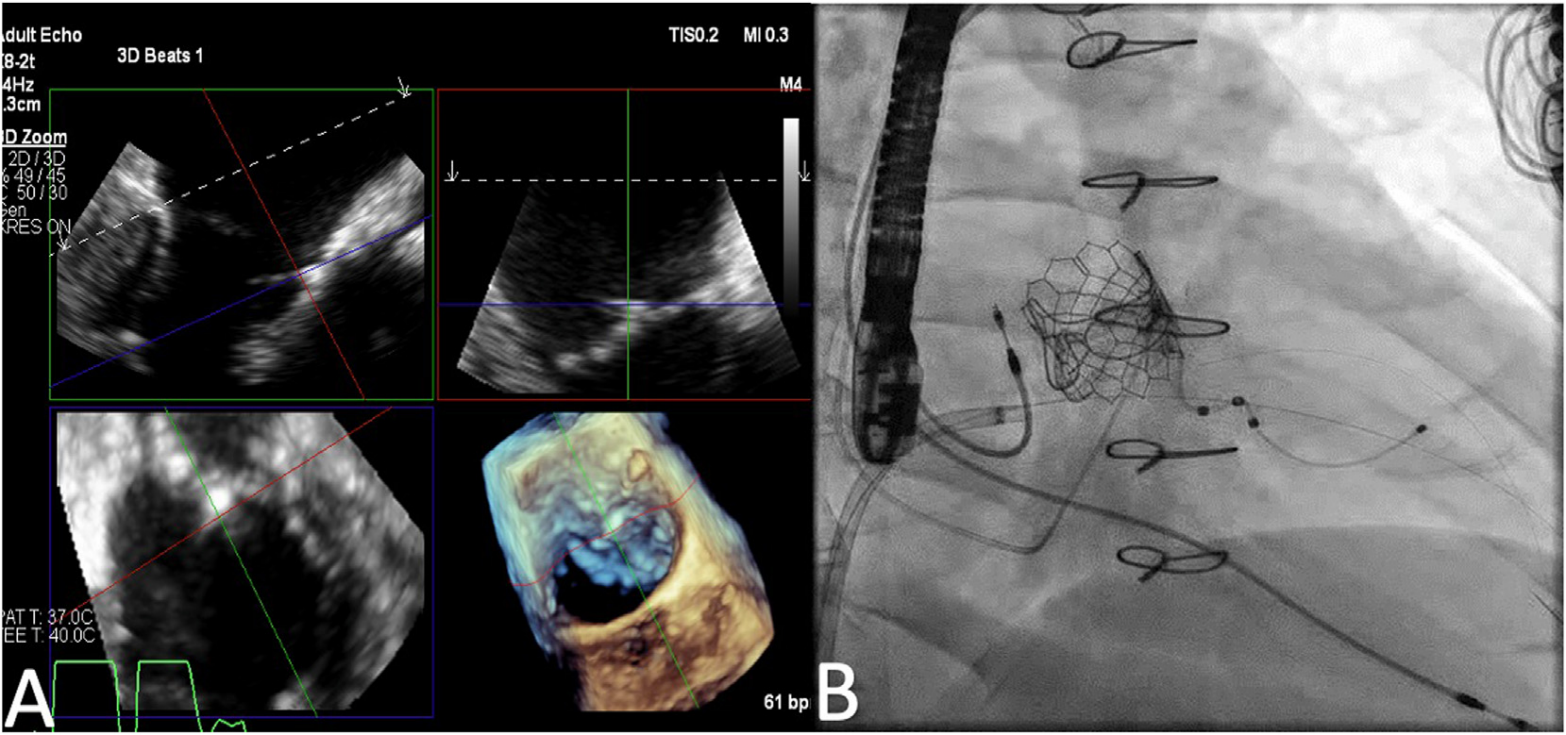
(A) Real-time 3D multiplanar reconstruction easily guided catheter/wire into perforation. (B) Fluoroscopic view showing transseptal access under real-time 3D multiplanar reconstruction transesophageal echocardiogram guidance, with anterior mitral leaflet perforation cannulated using 2 guidewires.
